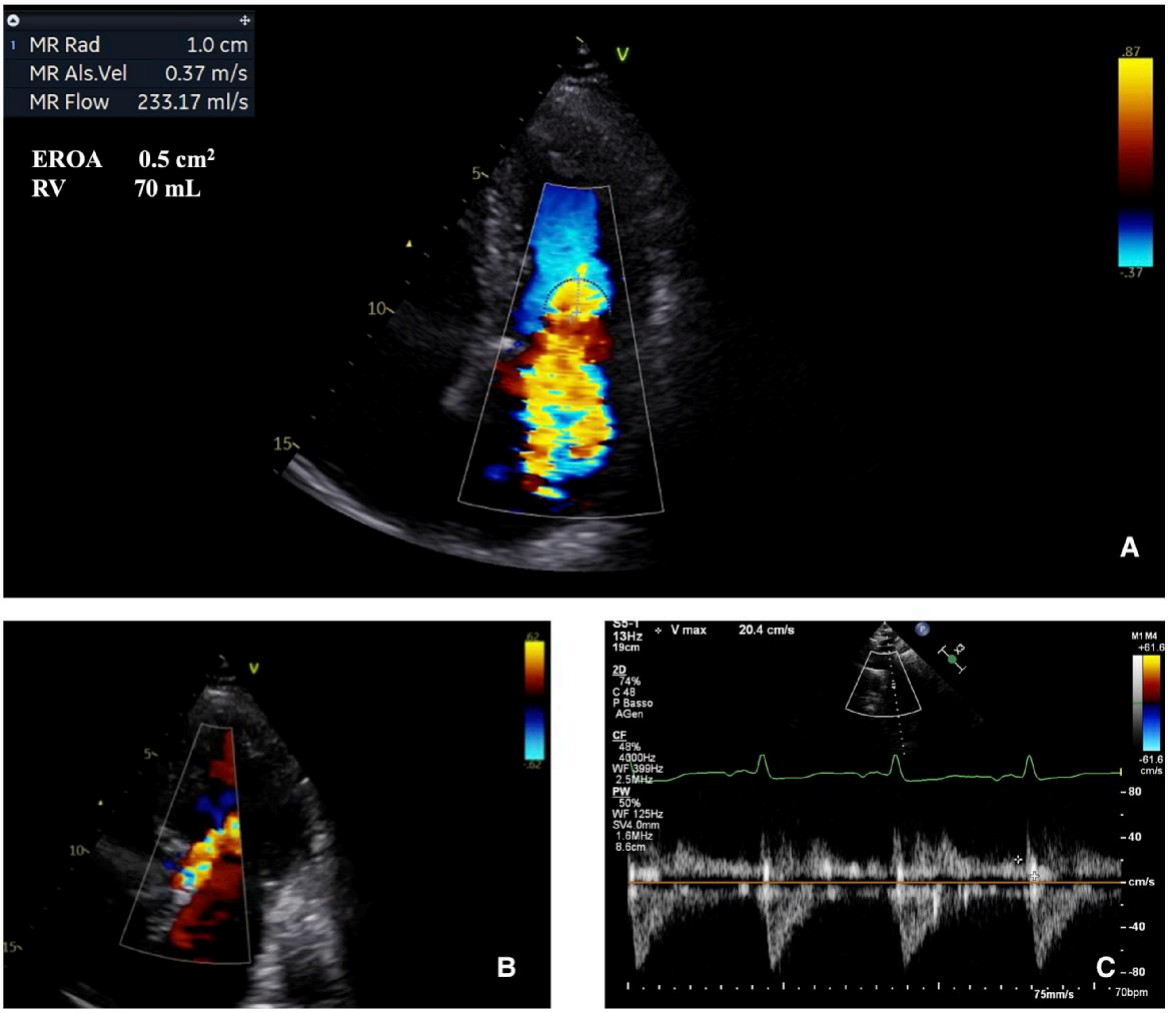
TTE on admission. (*A*) Evaluation of MR effective orifice area (EROA) and regurgitant volume (RV) using PISA method. (*B*) Color-Doppler evaluation of AR. (*C*) Pulsed-wave Doppler showing significant diastolic flow reverse in descending aorta.
